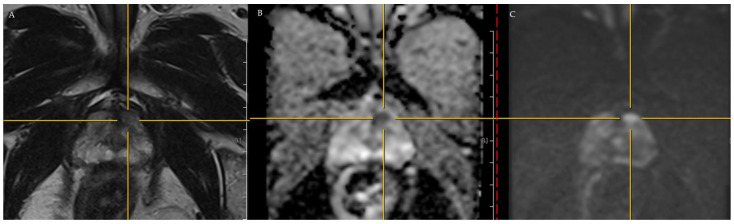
3T mpMRI assessment of the prostate. There was a PI-RADS version 2 score 4 lesion at the left anterior peripheral zone. (A) T2-weighted image. (B) Apparent diffusion coefficient. (C) Diffusion-weighted imaging.
